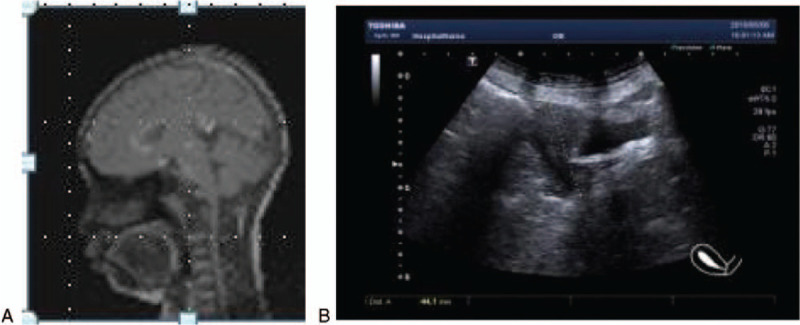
(A) Pituitary-enhanced MRI indicated partially empty sella. (B) A small uterus was detected, and both ovaries could not be identified on pelvis ultrasonograph.MRI = magnetic resonance imaging.
